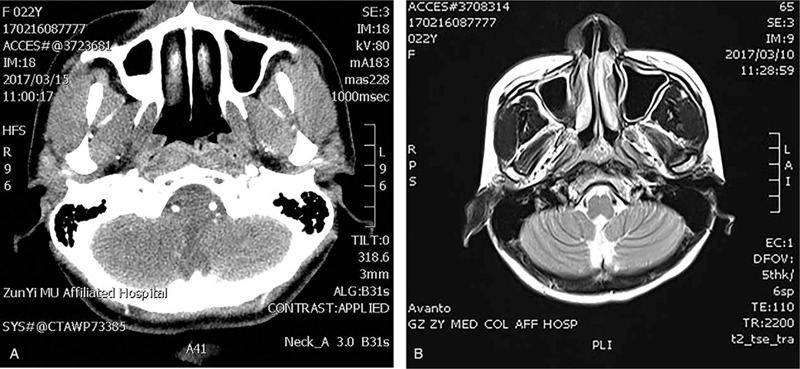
Computed tomography (CT) imaging and enhanced scanning of the head and neck confirmed a soft mass on the posterior of the nasopharynx (A). The tumor exhibited weak contrast enhancement (B).
